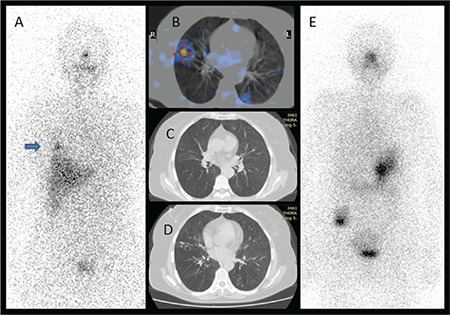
There was I-131 uptake at the lower zone of the right hemithorax on the anterior image of post-therapeutic I-131 whole body scan (A, arrow). The focal activity accumulation in the lung parenchyma on fused single photon emission computed tomography/computed tomography (CT) image (B), and findings on CT images (C, D) were concordant with bronchiectasis. The diagnostic I-131 scan (E) did not reveal any pathologic uptake and the serum thyroglobulin level was low (<0.2 ng/mL)
